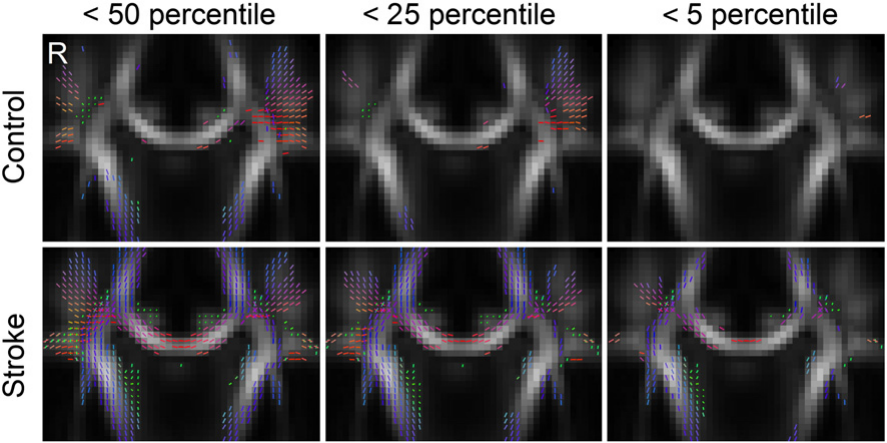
Diffusion MRI connectometry applied to a normal subject and to a patient with chronic stroke. The local tract orientations with diffusion quantities lower than 50th, 25th, and 5th percentile ranks were plotted with directional color coding. Both the control and the patient showed abundant local tract orientations with percentile ranks lower than 50, although very few local tract orientations with a percentile lower than 5 remained in the normal subject. The stroke patient displayed many fiber tracts affected by the stroke in the right internal capsule and right corpus callosum, where the local tract orientations were lower than the 5th percentile.
